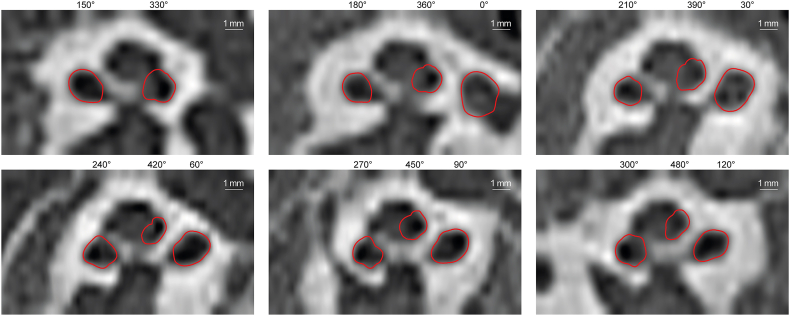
Overlay of segmented cochlear contours on clinical CT scans from the round window to 480° in 30° steps. The segmentation was successful with expected deviations in the second turn, especially at the inner and top walls where visual validation is challenging.
